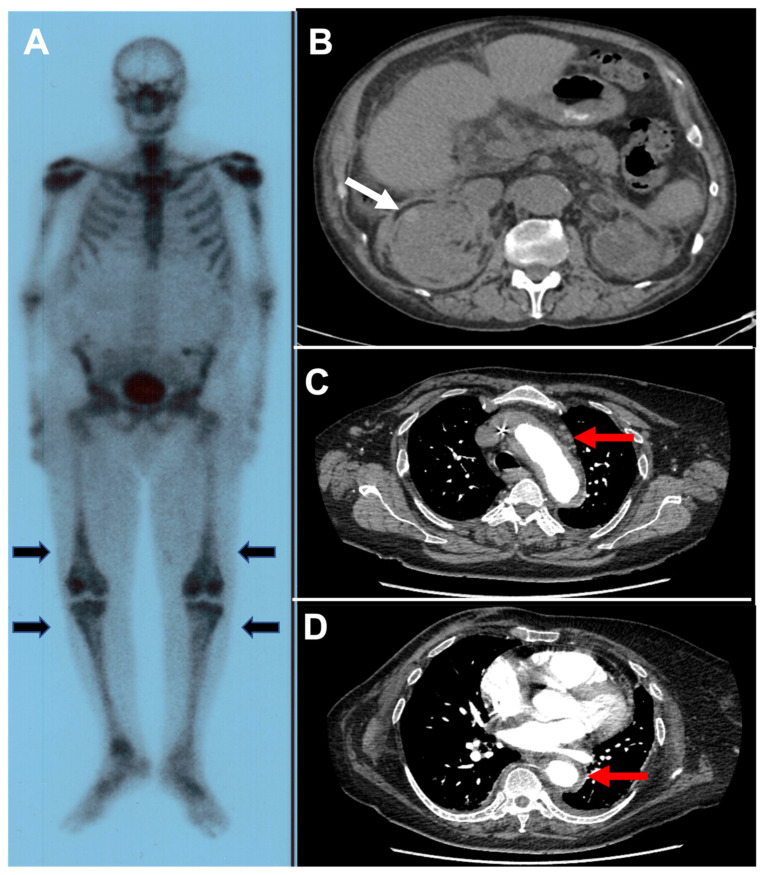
Erdheim Chester disease (ECD). Panel (A): Uptake of the tracer by long bones on the ^99^Technecium bone scintigraphy (black arrows). Panel (B): CT-scan showing a symmetrical infiltration of the perirenal fat and of the perirenal fascia taking the appearance of “hairy kidneys” (white arrow). Panel (C,D): angio-CT scan showing a peri-aortitis (coated aorta) in a patient with ECD (red arrows).
